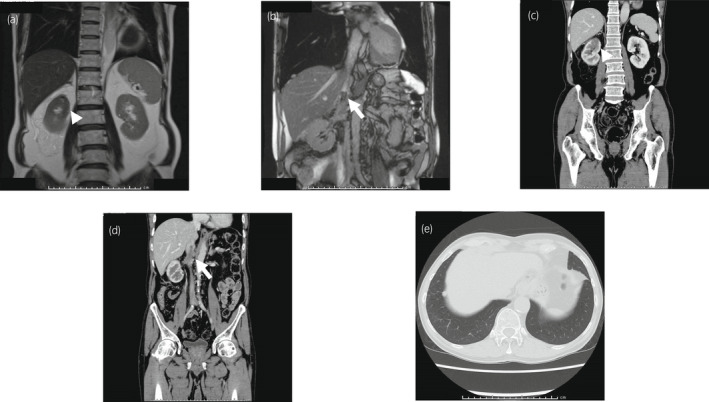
Images of the renal tumor and IVCTT after four cycles of Nivo‐Ipi and four cycles of nivolumab monotherapy. (a, b) T2‐weighted coronary images of MRI. (c, d) Images of contrast‐enhanced CT. (e) A CT image of the pulmonary metastatic lesion. The renal mass and IVCTT had regressed and multiple pulmonary masses had diminished, except for one lesion.
